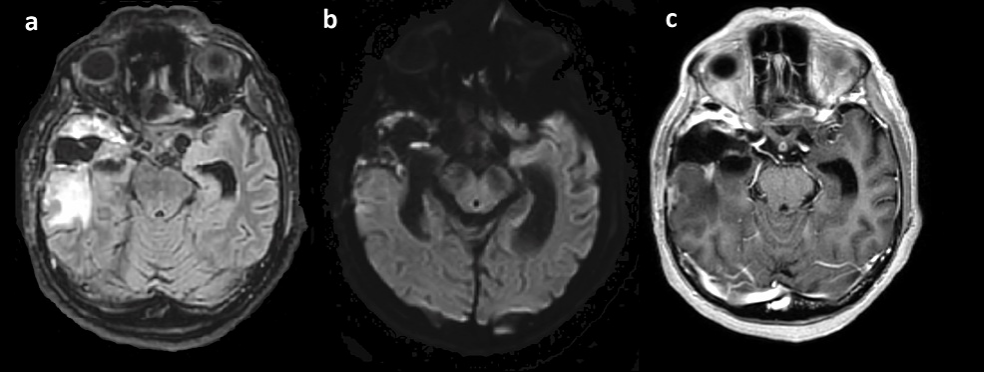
One-month follow-up brain magnetic resonance imaging (MRI) (a) Axial T2 weighted sequence showing neurosurgical residuals one month after abscess evacuation; (b) DWI-sb1000 and (c) axial contrast-enhanced 3D MPRAGE sequence showing no abscess residuals. DWI: Diffusion-weighted imaging; 3D MPRAGE: three-dimensional magnetization-prepared rapid gradient-echo
